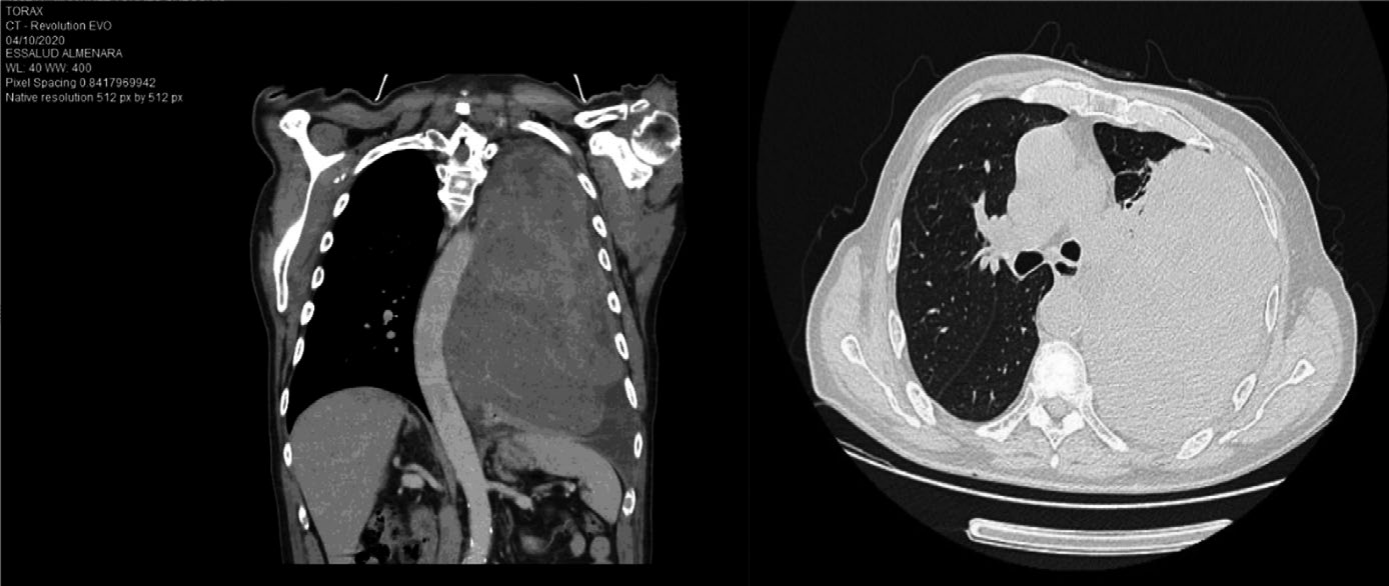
Coronal section chest CT scan shows a mass with a neo‐proliferative appearance of the left lung measuring 24.9 cm × 15.8 cm × 18.9 cm, associated with ipsilateral laminar pleural effusion and mass effect on the contralateral mediastinal structures
